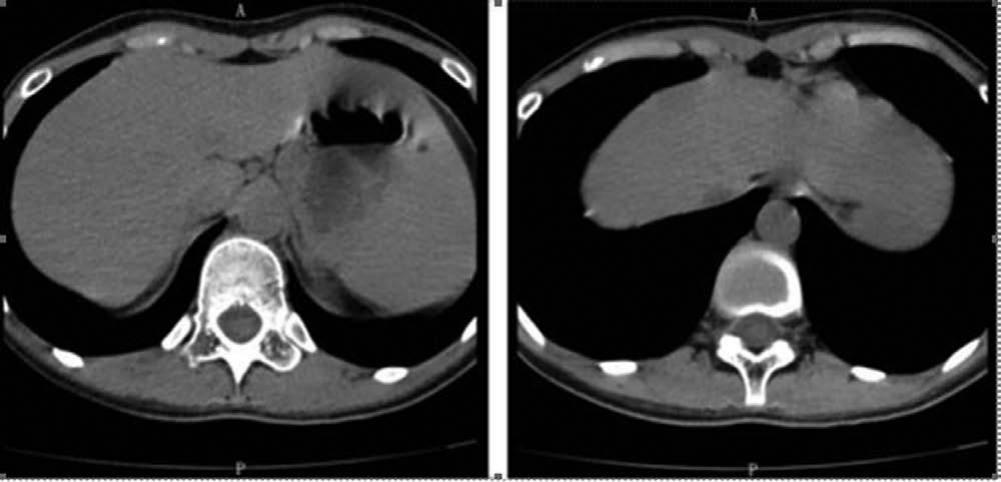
Bilateral adrenal CT scan with kidney findings showing probabilities of papillae caused by calcium milk deposition. adrenal shows no abnormalities; however, a significant amount of calcium milk deposit affects both kidneys. CT = computerized tomography.
